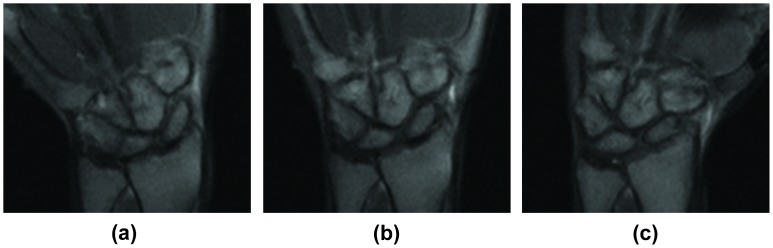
Active-MRI of the wrist during active ulnar-radial deviation. Snapshots of the coronal images of the wrist in the ulnar deviation (a), neutral (b) and radial deviation (c) positions during the continuous radial-ulnar deviation maneuver. SL interval and ulnar variance (see Figure 2 for these definitions) were measured from the resulting images.
